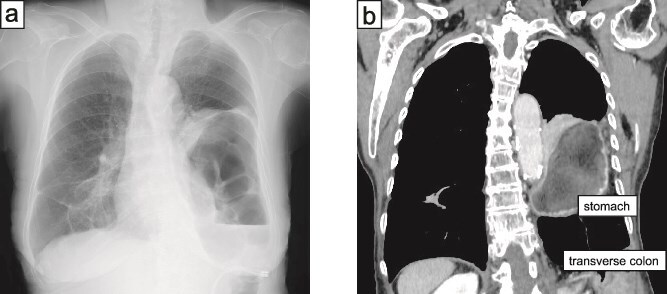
Postoperative imaging findings. (a) Chest radiograph demonstrating elevation of the left hemidiaphragm. (b) Contrast-enhanced CT showing intrathoracic herniation of the stomach (cardia to proximal body) and part of the transverse colon through the diaphragmatic defect.
